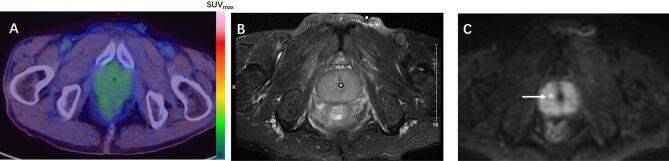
A 73-year-old patient with a PSA level of 4.29 ng/ml. The biopsy results of the two punctures showed chronic granulomatous inflammation of the prostate tissue. After 3 months of follow-up, the PSA fluctuations ranged from 3.52 to 5.39 ng/ml, suggesting benign lesions. ^68^Ga-PSMA-617 PET/CT (A) showed no significant change in the uptake of ^68^Ga-PSMA-617 in the prostate, with an SUVmax of 3.0, consistent with benign prostatic lesions. In mpMRI, there was no obvious abnormal signal on T2W (B), but DWI (C) suggested indicated a spot abnormal signal in the center of the right side of the prostate (indicated by the arrow), suggesting PCa, but it was inconsistent with the pathological results.
